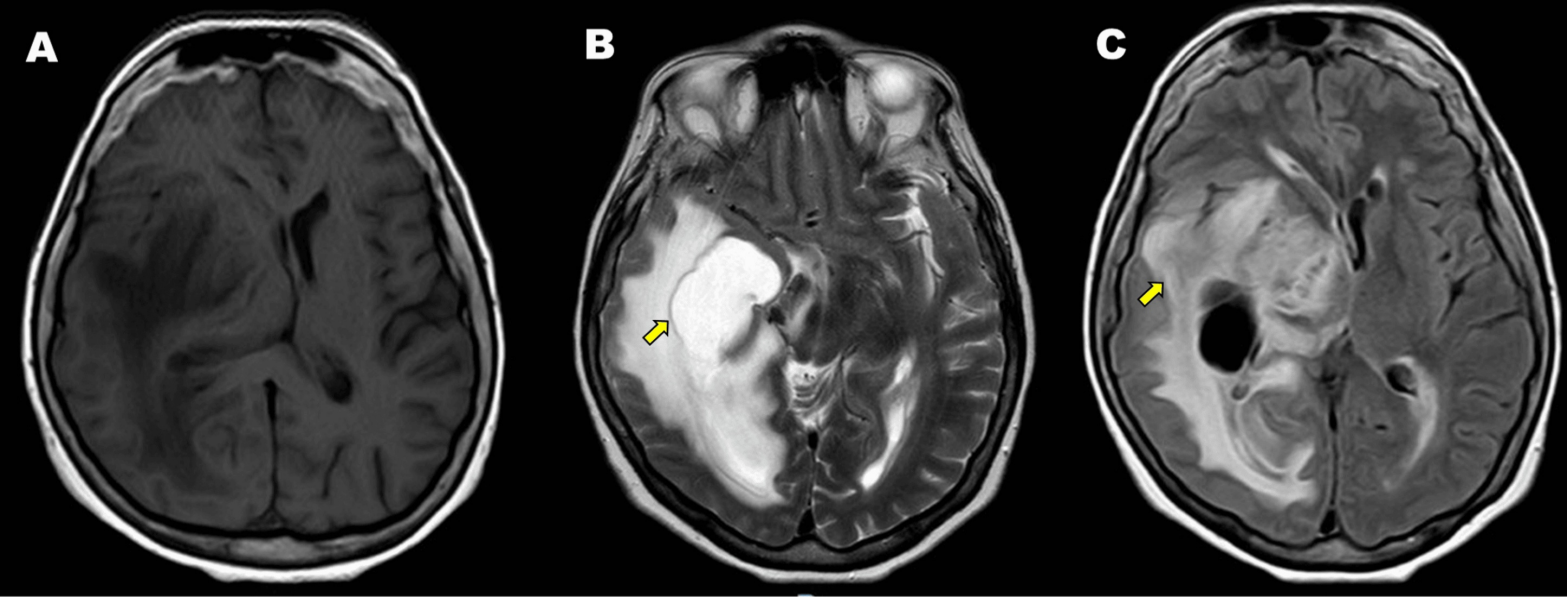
Brain MRI. Intra-axial lesion appearing hypointense on T1-weighted sequence (A), hyperintense on T2-weighted sequence (B), and hypointense on FLAIR sequence (arrow) (C), located in the right parieto-occipital region. The lesion measures approximately 4.8 cm in length and 2.6 cm in thickness, with contralateral midline shift and associated vasogenic edema (arrow) MRI: magnetic resonance imaging; FLAIR: fluid-attenuated inversion recovery
